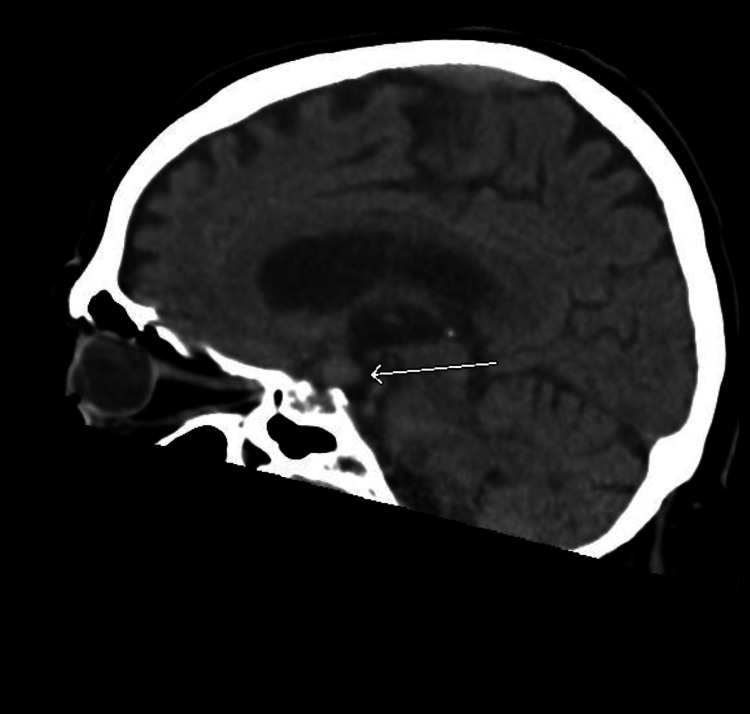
CT of the brain (sagittal view) showing a suprasellar mass
